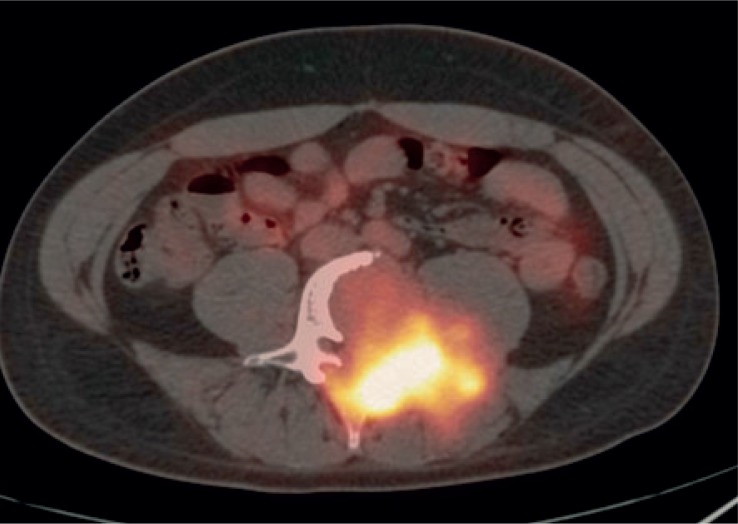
29 year old male with unusually aggressive extraskeletal myxoid chondrosarcoma presenting in the calf. Axial FDG-PET/CT demonstrating significant FDG uptake (SUV_max_ 7.4) in bone metastasis in a patient with high density tumor burden.
